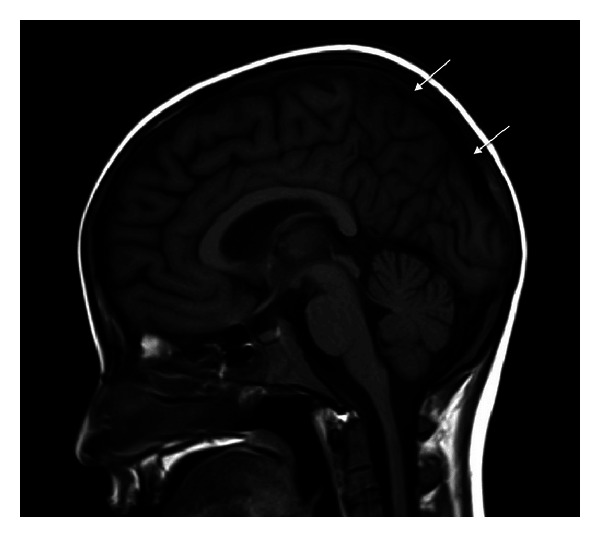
Sagittal T1-weighted MRI reveals normal flow signal indicating total resolution of superior sagittal sinus thrombosis (arrows).
